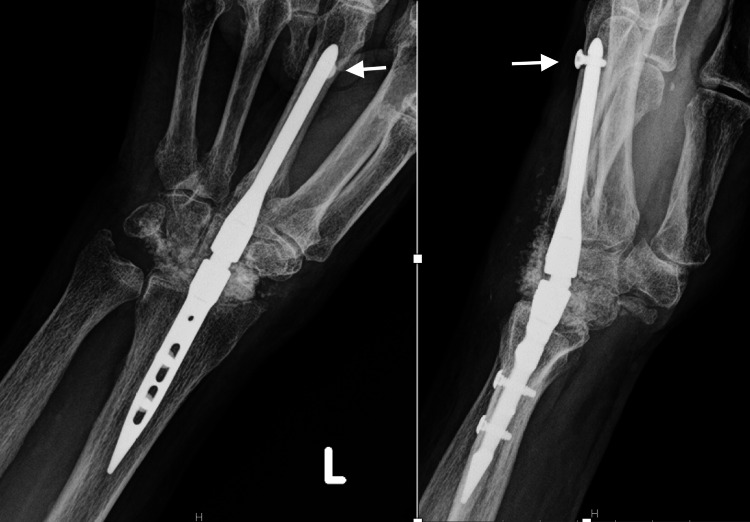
Immediate postoperative radiographs of Case 1 Posteroanterior and lateral views after total wrist arthrodesis using IMPLATE intramedullary nail and proximal row carpectomy. The white arrows show the proper positioning of the distal screw.
